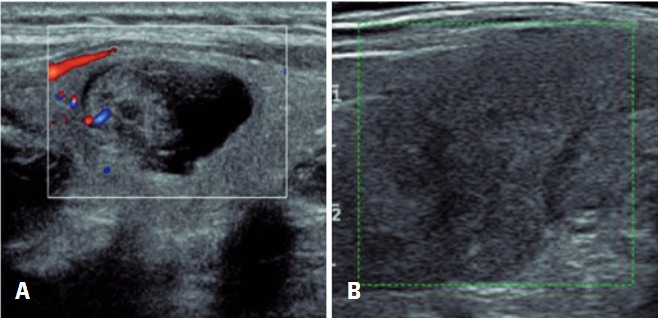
Thyroid ultrasound with unexpected results. (A) Nodule classified as TI-RADS 2 that showed malignancy in cytological examination. (B) Nodule classified as TI-RADS 5 with granulomatous thyroiditis in cytological examination (Bethesda II)
